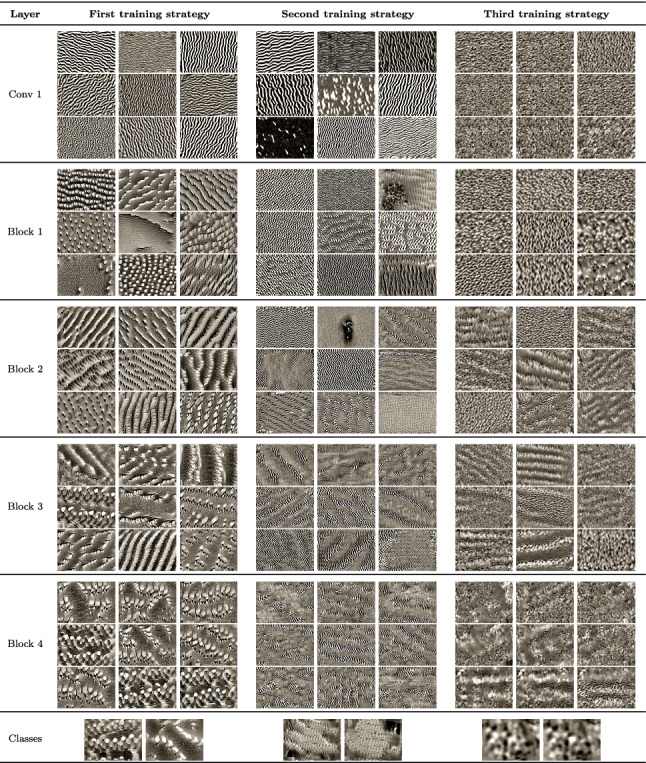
Feature visualization for single channels in CovidResNet trained on the SARS-CoV-2 CT-scan dataset with different training strategies. Best viewed in color with zoom
